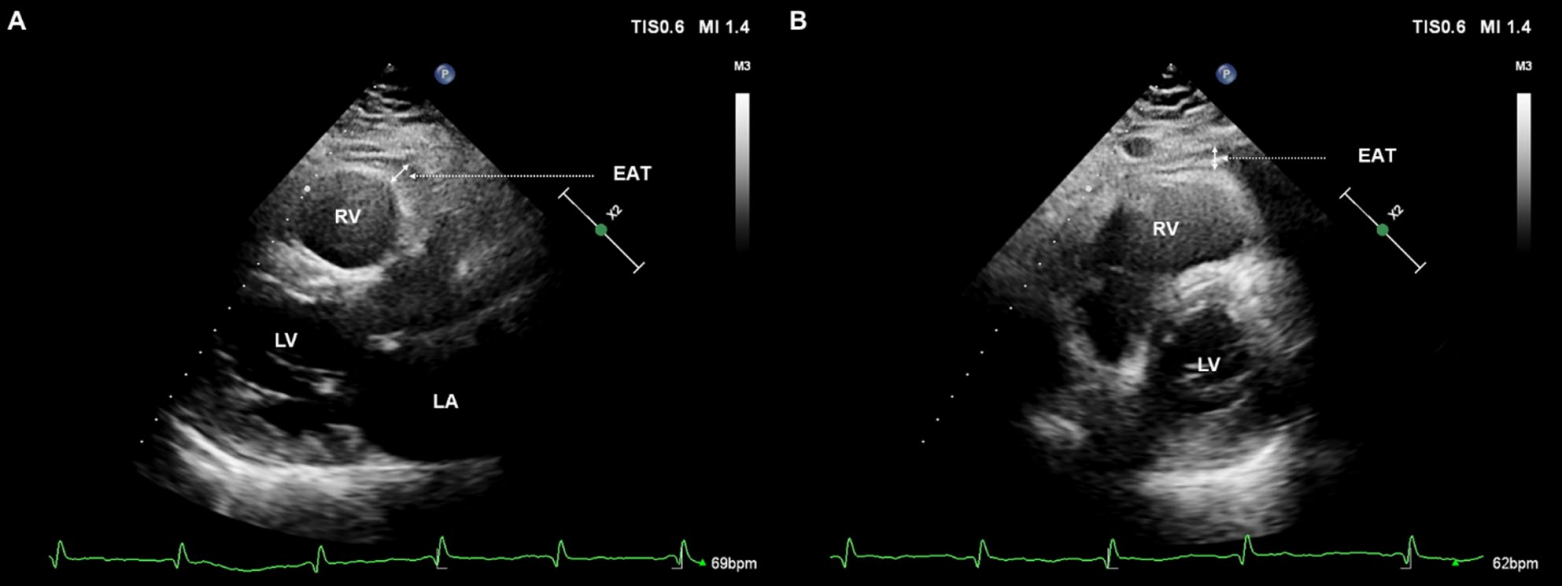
Echocardiographic measurement of EAT thickness on the free wall of the RV at end-systole. A Parasternal long-axis view. B Parasternal short-axis view. EAT thickness (white solid line) was identified as the echo-free space between the outer wall of the myocardium and the visceral layer of the pericardium. EAT, epicardial adipose tissue; RV, right ventricle; LV, left ventricle; LA, left atrium
